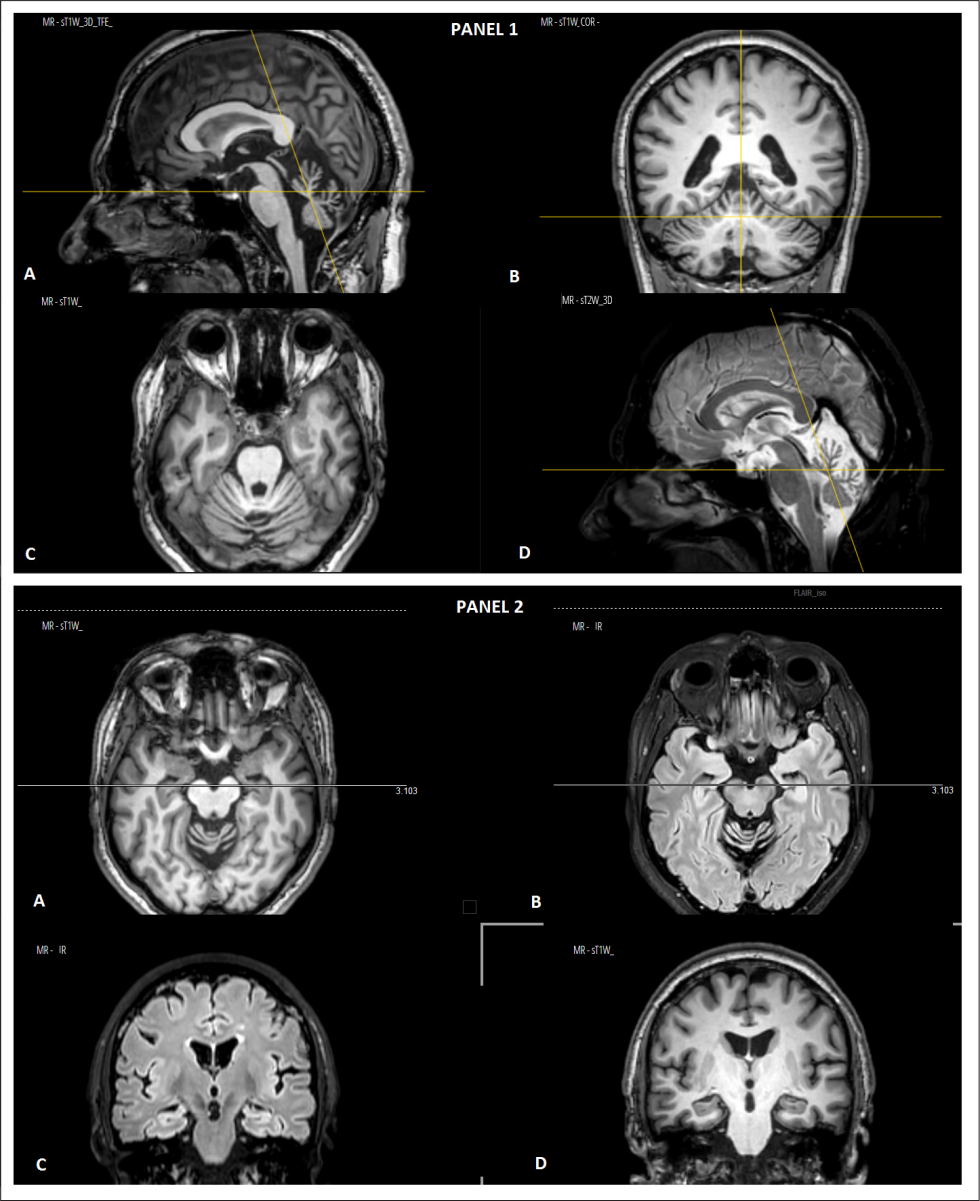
Panel 1. Brain MRI of the mother of the first family’s proband, showing prominent cerebellar atrophy, especially in the vermis. (A–C) T1-weighted images centered on the cerebellum on sagittal (A), coronal (B) and axial (C) planes; D) sagittal T2-weighted image. Panel 2. MRI study showing moderate bilateral atrophy of the body of the hippocampus. (A, B) Axial images centered on the hippocampi on T1-weighted (A, D) and IR-w (B, C) images.
